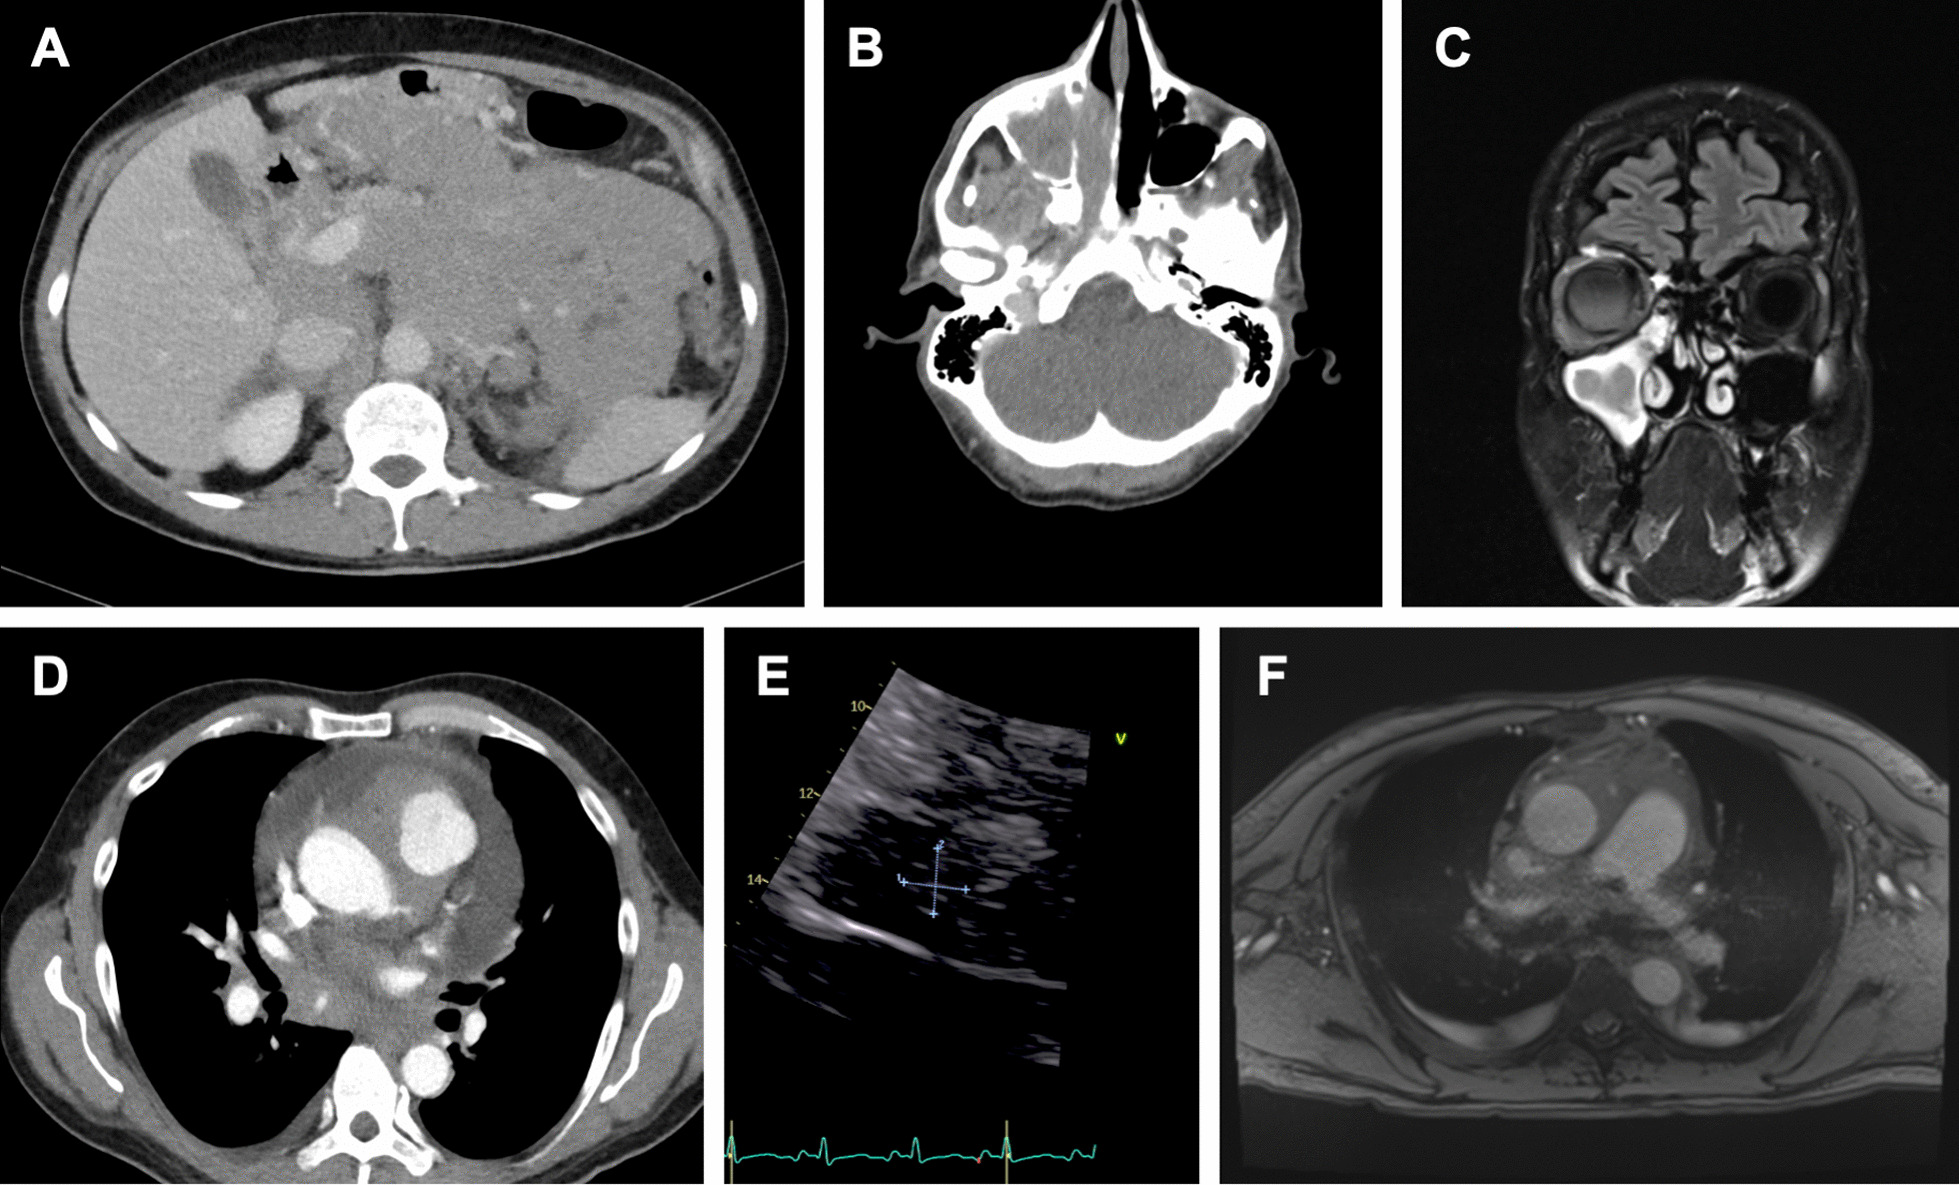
CT and MR imaging reveal extensive BL manifestations. A Contrast-enhanced CT, large abdominal manifestation with infiltration of pancreas and mesenteric root. B and C Contrast-enhanced skull CT and MRI, destruction of posterior wall of right maxillary sinus by BL manifestation. D Contrast-enhanced chest CT, mediastinal manifestation with infiltration of supraaortic vessels. E TTE revealing a solid mass of 2.2 × 1.3 cm of the right atrium. F Cardiac MRI: mediastinal and pericardial mass with infiltration of pericardium and bilateral pleural effusion
